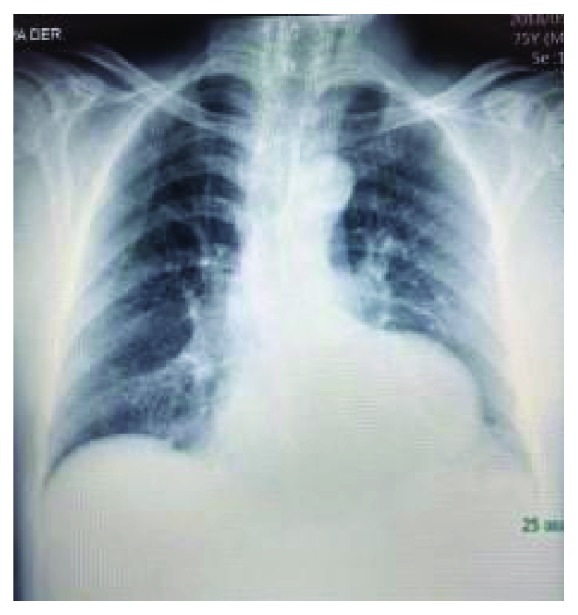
Posteroanterior thoracic radiography denotes cardiomegaly imparted mostly by the low left cardiac bulging.
